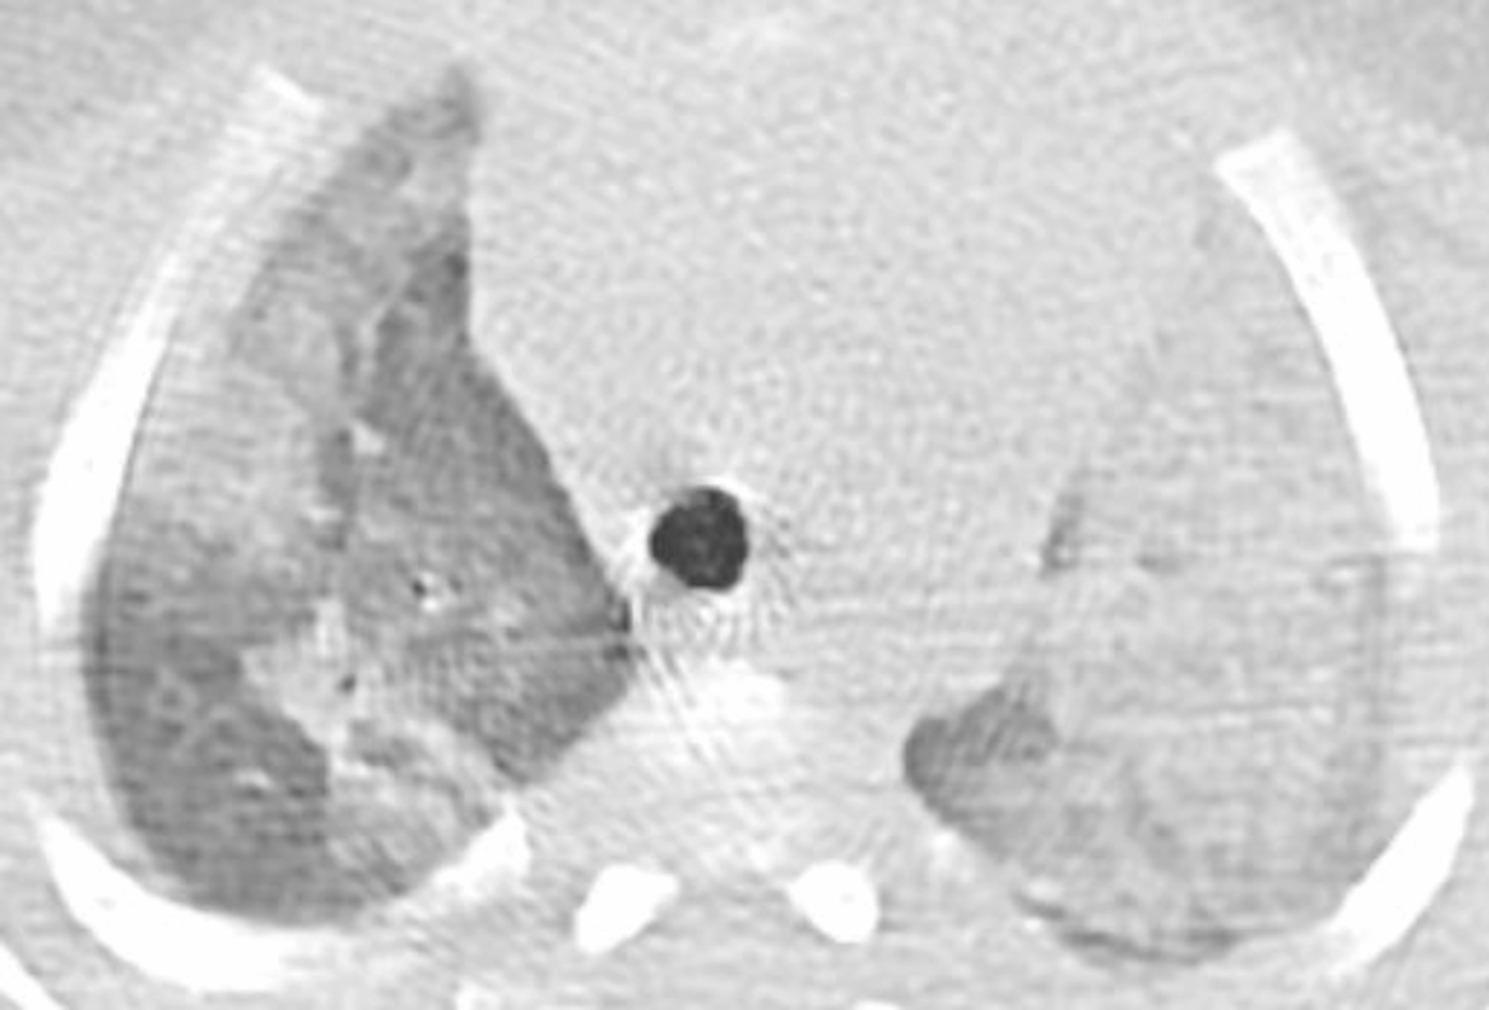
CT at the level of trachea
